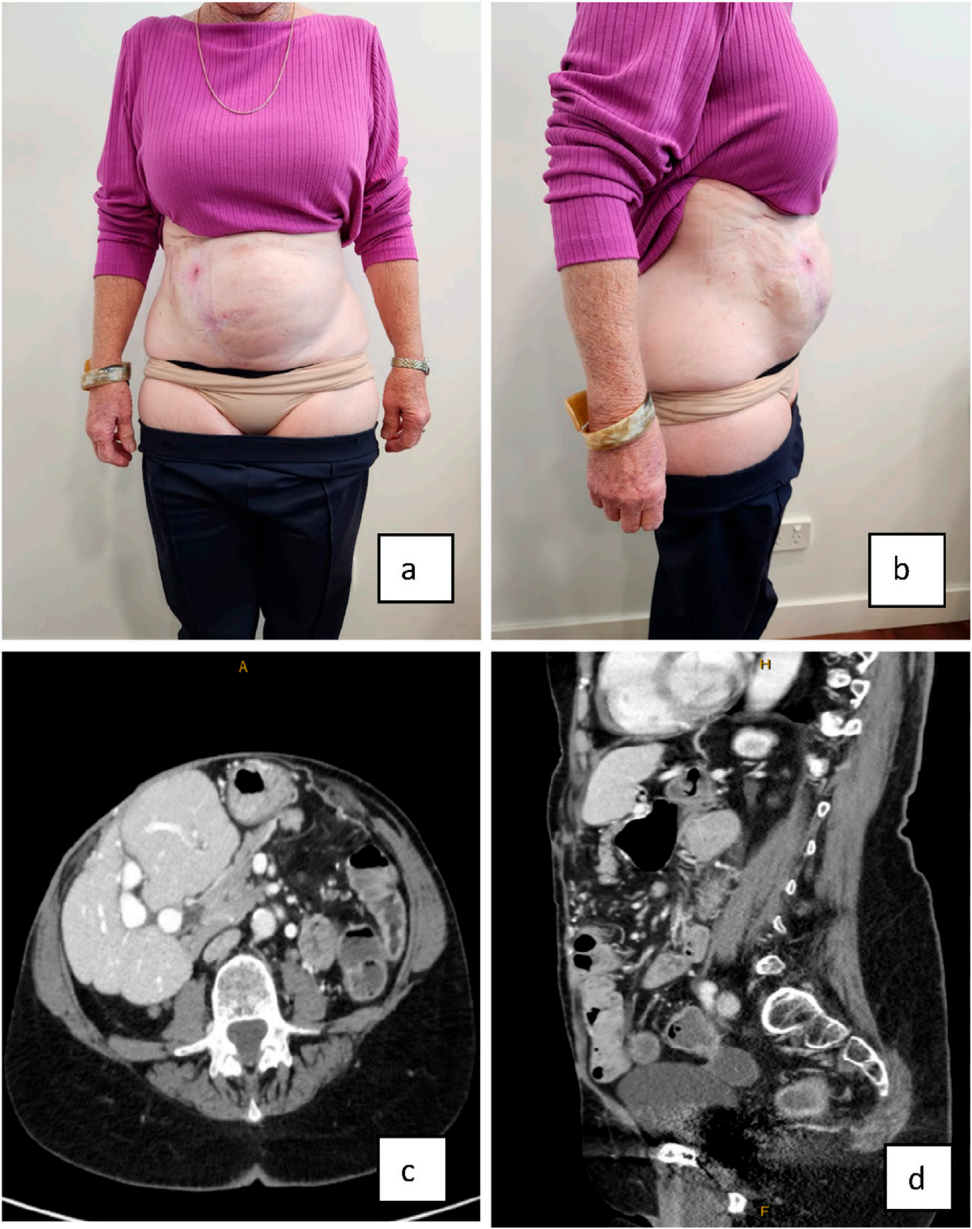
(A,B) Final result of abdominal wall closure three and a half years later clinical appearance and imaging. (C,D) Axial and sagittal CT scans demonstrating that the loss of domain evident preoperatively, has not recurred and the oblique muscles have remained elongated.
